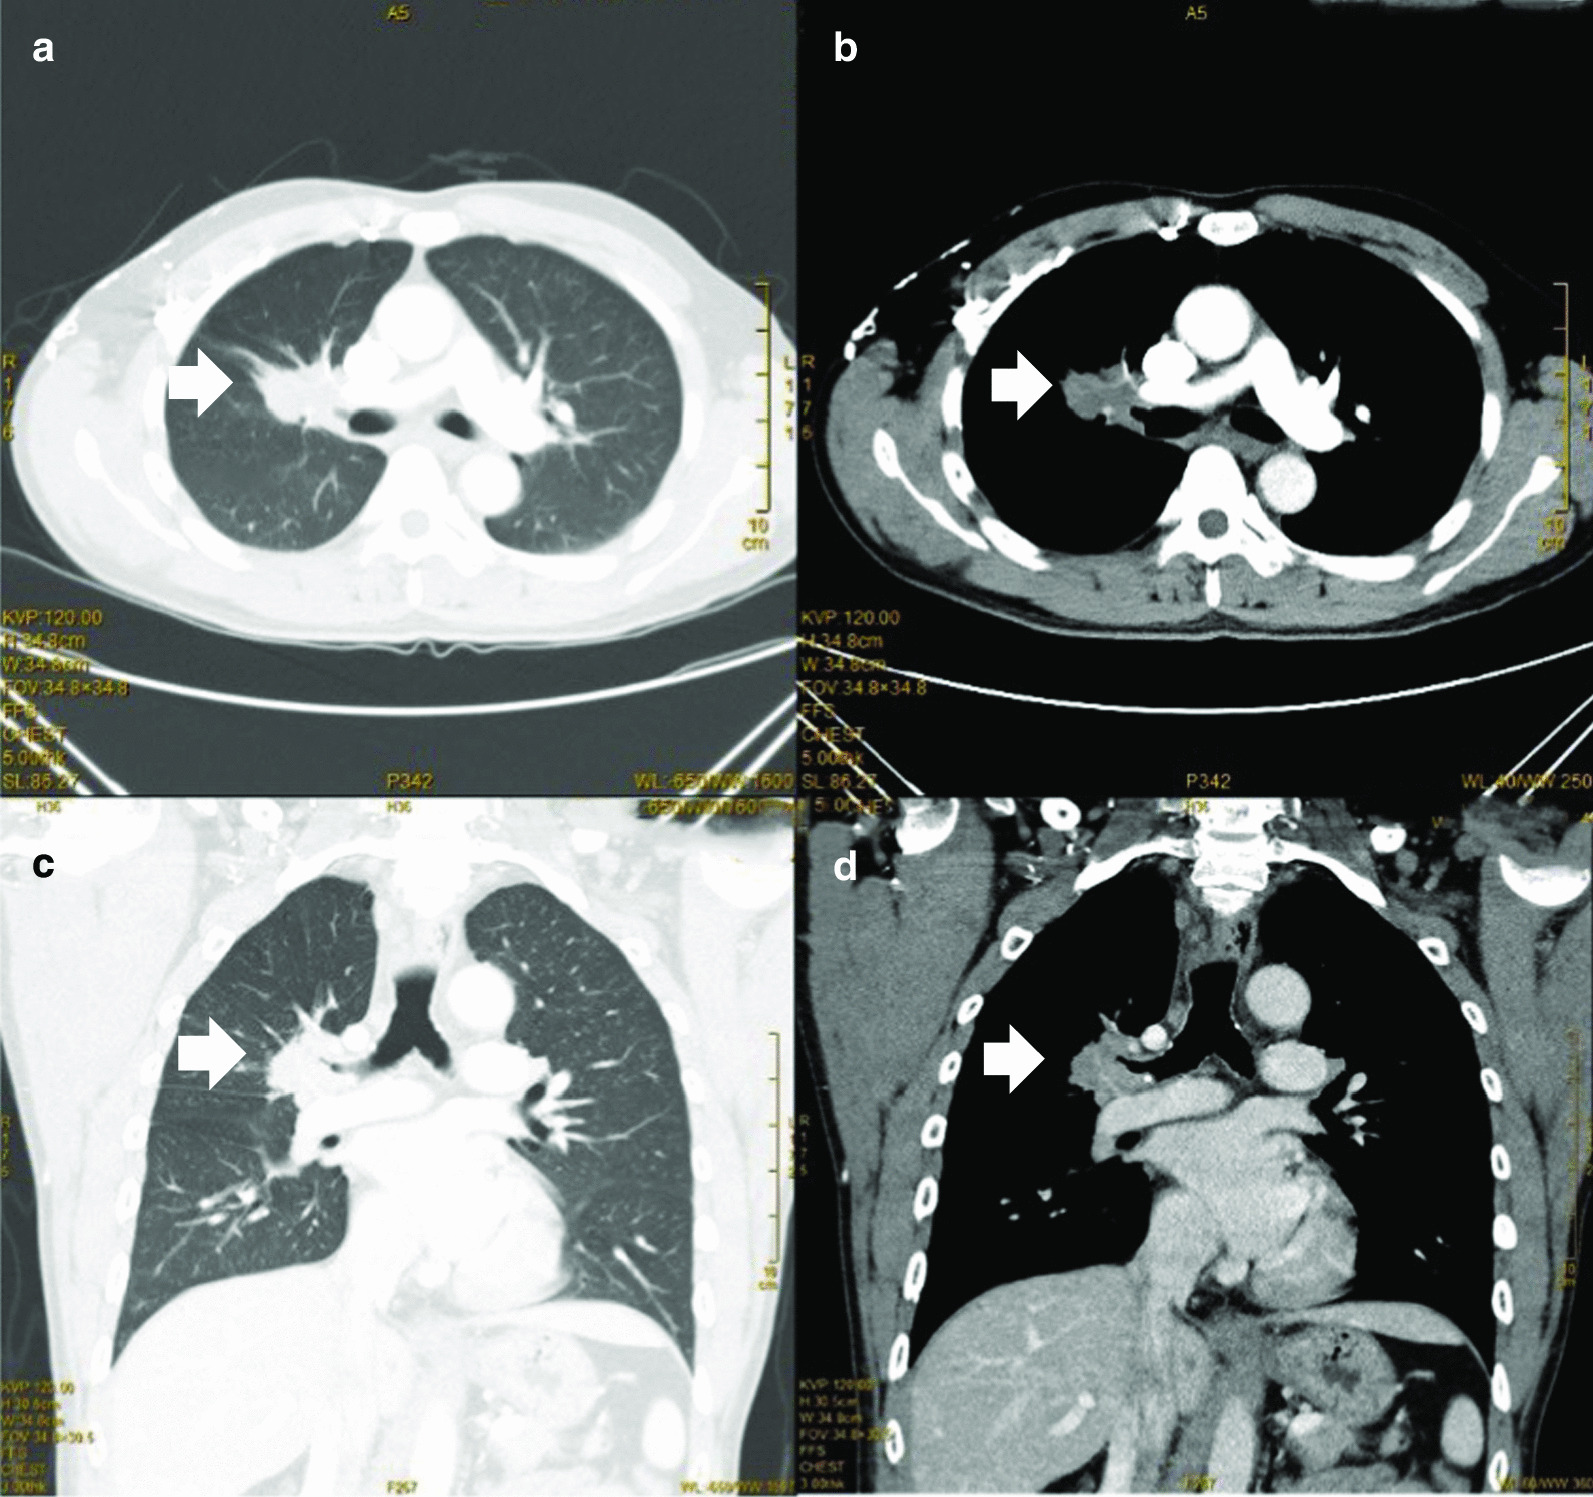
Chest CT of right central lung cancer. a. Cross-sectional, lung window showed right central lung cancer. b. Mediastinal window, tumor invaded right main bronchus and right pulmonary artery trunk. c d. Frontal plane, right central lung cancer invade right main bronchus and right pulmonary artery trunk
